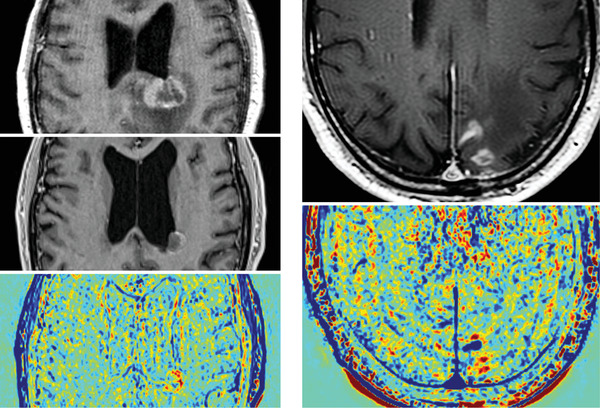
In the case of a 62‐year‐old man with biopsy confirmed primary diffuse large B‐cell lymphoma of the CNS treated with methotrexate, cytarabine, thiotepa and rituximab (MATRix) chemoimmunotherapy and busulphan/thiotepa autograft, follow‐up contrast enhanced magnetic resonance imaging (CE‐MRI) demonstrated ongoing peripheral enhancement at the site of original lesion (middle left). Here we show that treatment response assessment maps (TRAMs) can be used to differentiate active disease from benign enhancement.
